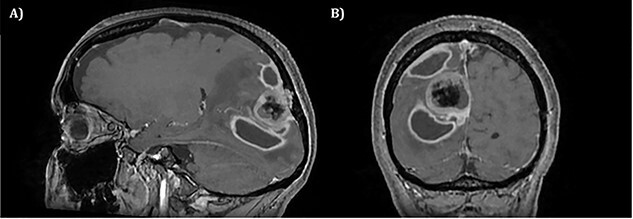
T1 weighted MRI postgadolinium enhancement in both sagittal (A) and coronal (B) views. There are two new rim-enhancing cystic lesions. A superior lesion of 31.4 mm as measured on the coronal reconstructed images and is abutting the adjacent dura of the right parietal lobe and is sited superior and lateral to the right occipital lesion. Another lesion sited inferior to the known right occipital lesion is the larger of the two lesions and has a septation within it. This measures approximately 34.4 mm on the coronal reconstructed images. On the sagittal images, it can be seen that the anterosuperior enhancing border of this lesion has a thicker and more ill-defined age. There is an adjacent vasogenic oedema.
